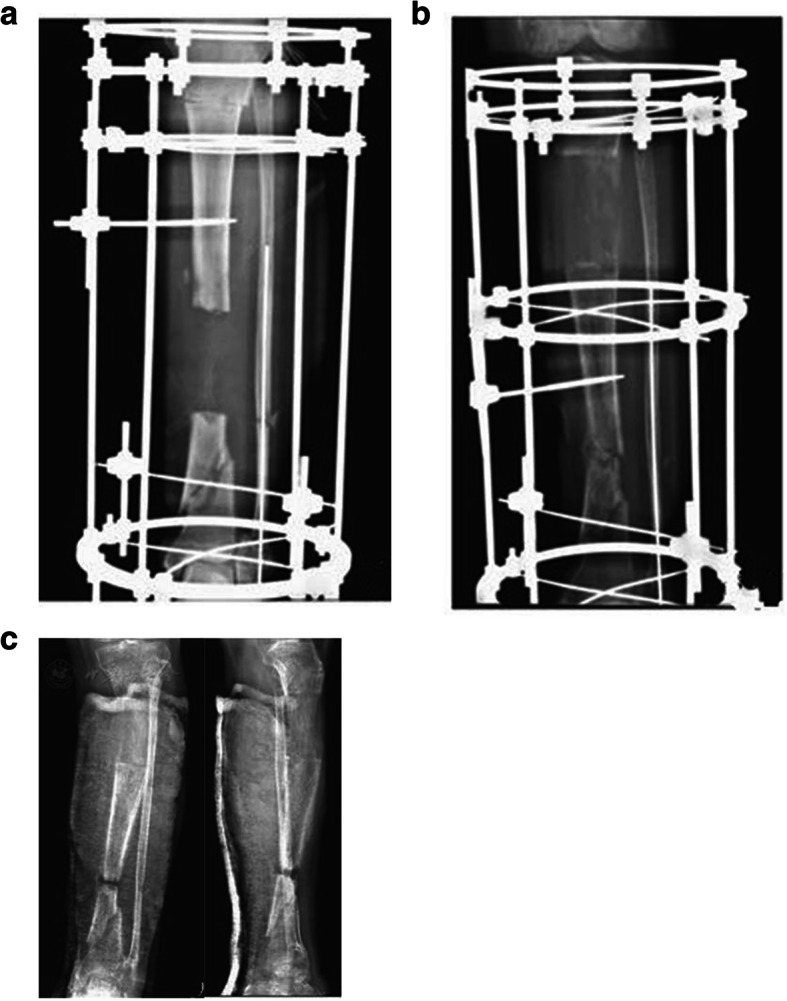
Patient with fracture and defect of tibia and fibula treated with bone transport (a,b), in whom the X-Ray showed the TBS retracted 4.0 mm in a timing of removal of 7 months and a time interval of 10 days (c)
